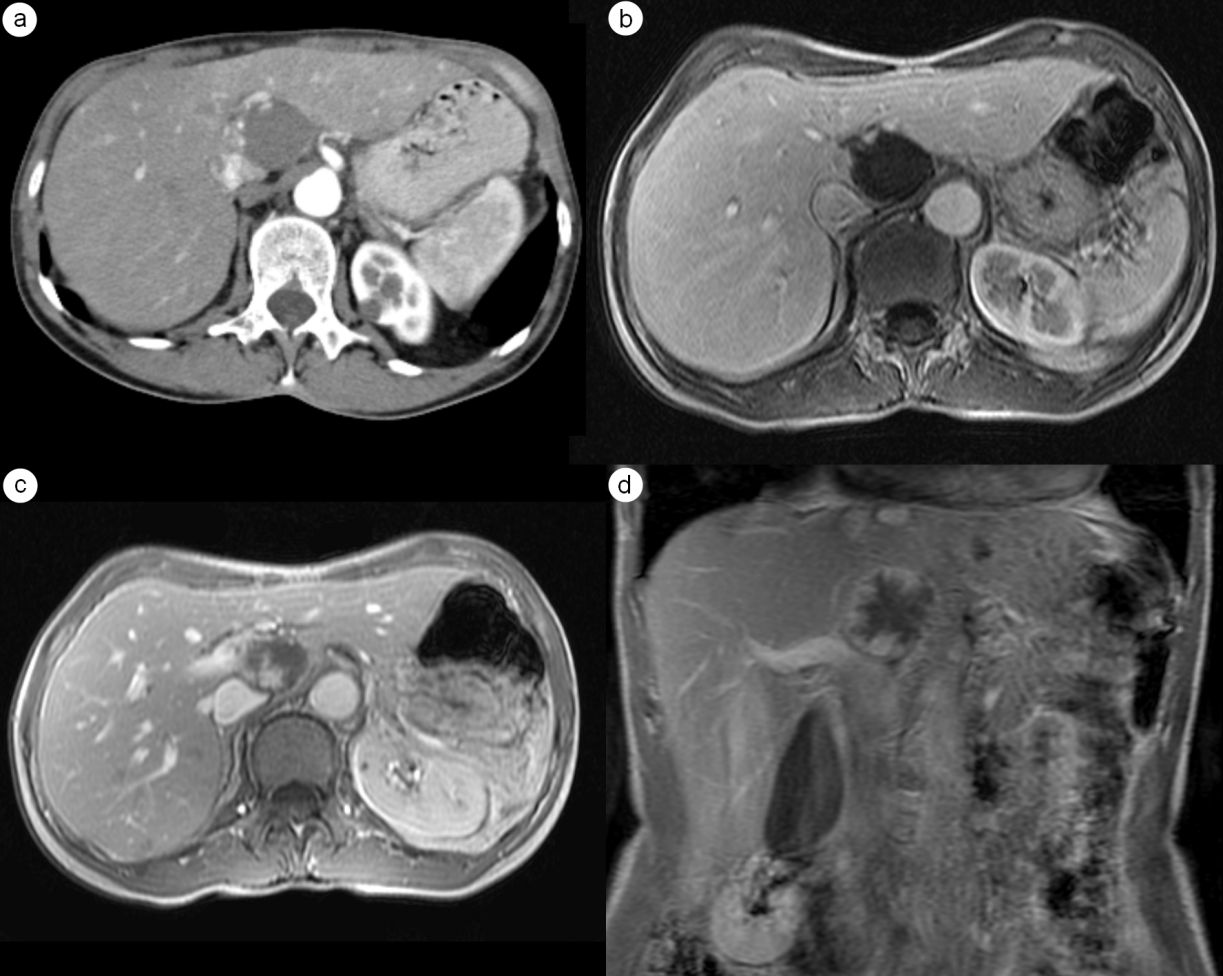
The same case of the hepatic hemangioma as in figure 2. HAP-CT image clearly visualizes globular type of enhancement (a). Globular pattern of enhancement is also visible in HAP - MR image (b). Progressive fill-in enhancement pattern can be observed in PVP (c) and EP (d) phases of MR study.
